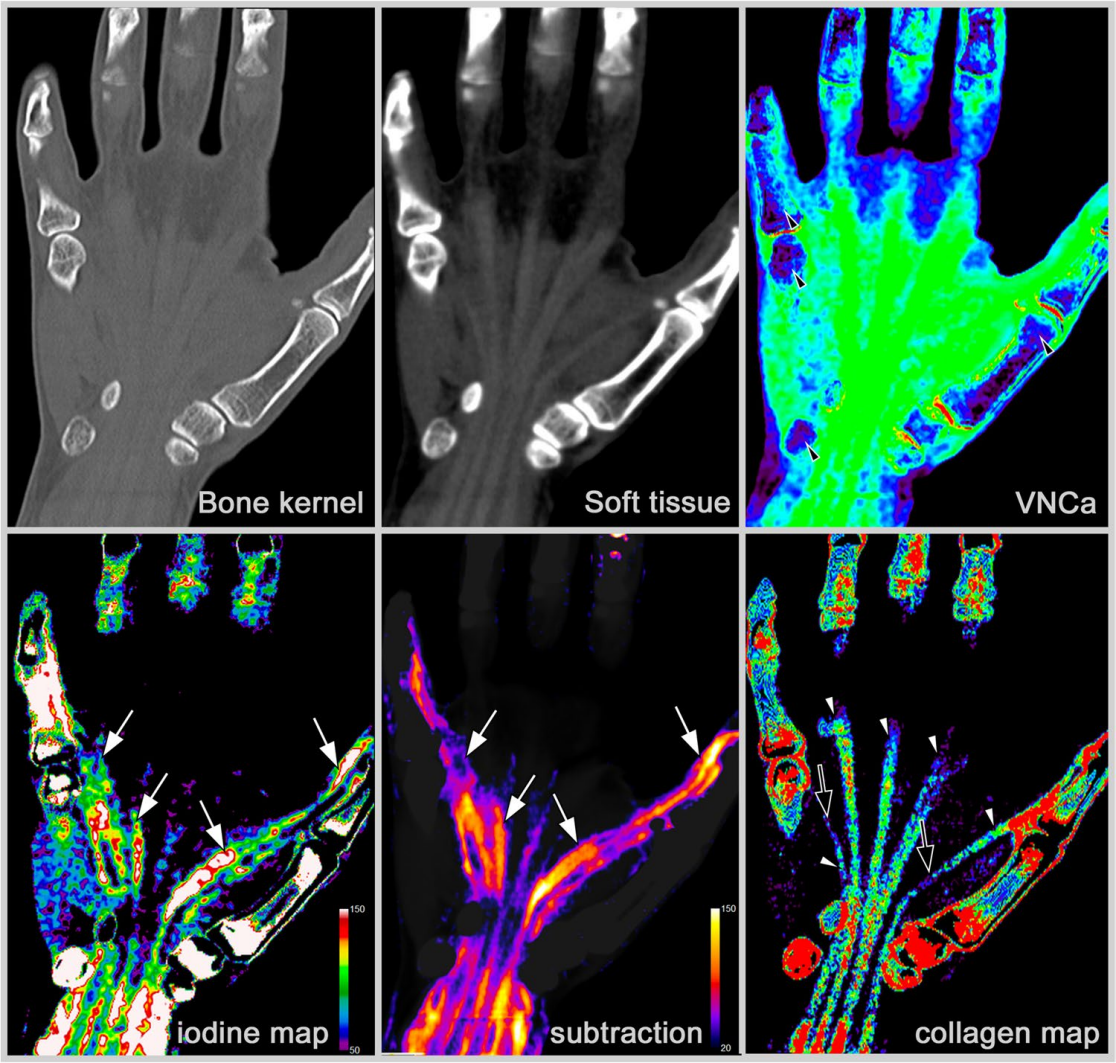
Reconstructions derived from contrast-enhanced DECT in a patient with rheumatoid arthritis. Presented are images in standard bone and soft-tissue kernel. Virtual non-calcium images (VNCa) show normal bone marrow (black arrowheads). Iodine maps show enhancement of the flexor tendons (white arrows); however, the inflammation is better depicted in CT subtraction (white arrows). Collagen maps show the flexor tendons (white arrowheads) with somewhat decreased collagen density in areas with marked inflammation (black arrows)
